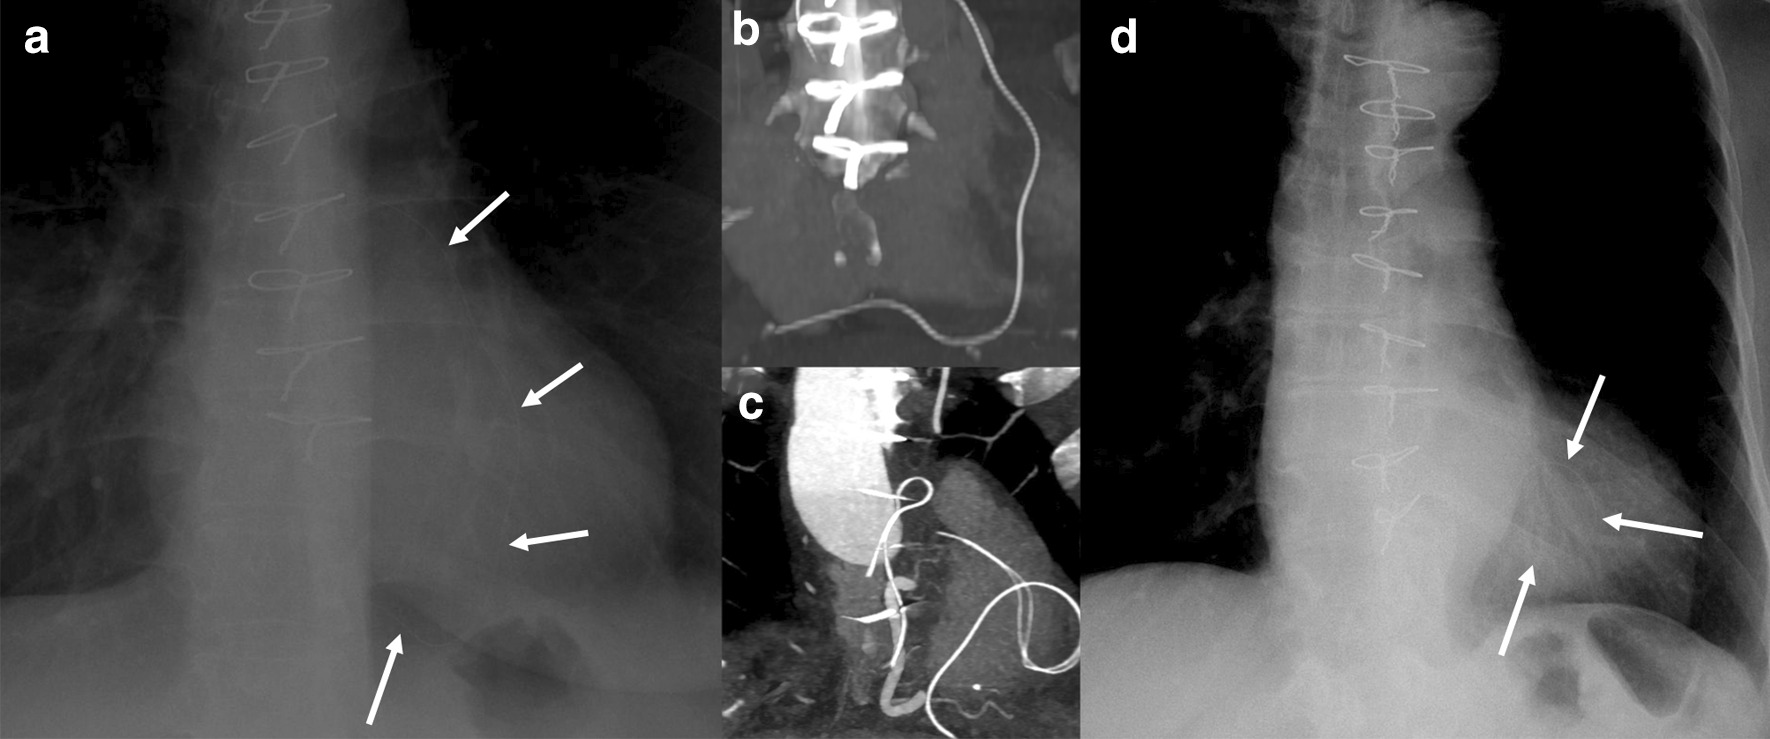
Representative chest x-ray (a, d) and maximum intensity projection images computed tomography (CT) (b, c) images of patients with retained temporary wires (C shaped configuration in a, b; looped configuration in c, d. Note the impaired visibility of the wires (white arrows) on the conventional chest X-Rays
